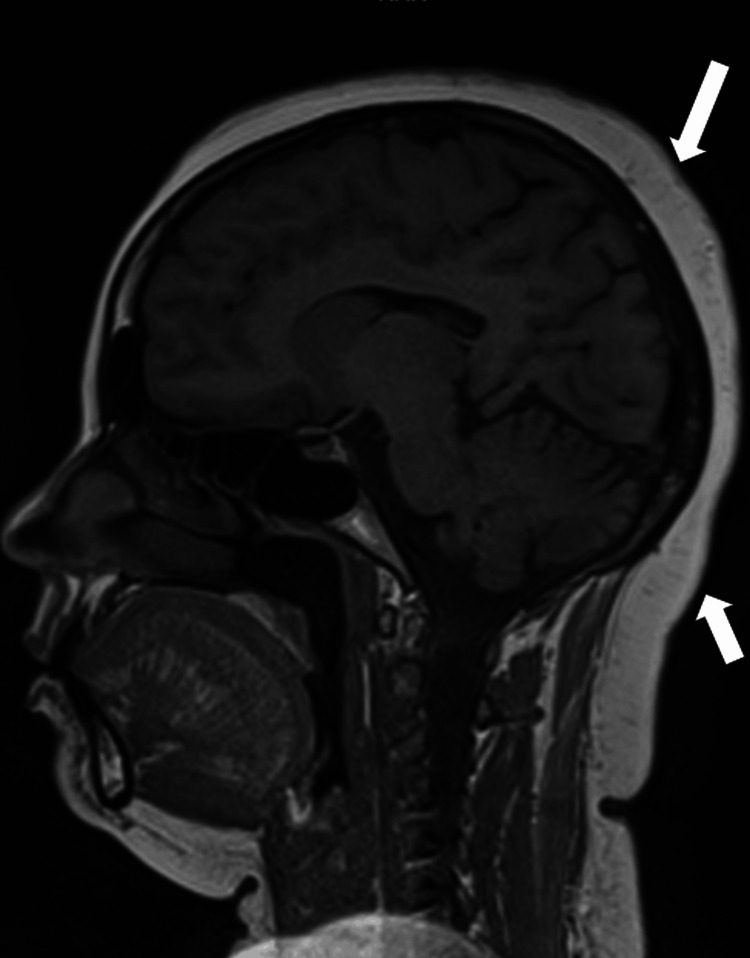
MRI brain (T1) showing scalp thickness of upto 12mm over vertex and occiput (arrows), with normal cortical findings. MRI: Magnetic resonance imaging
